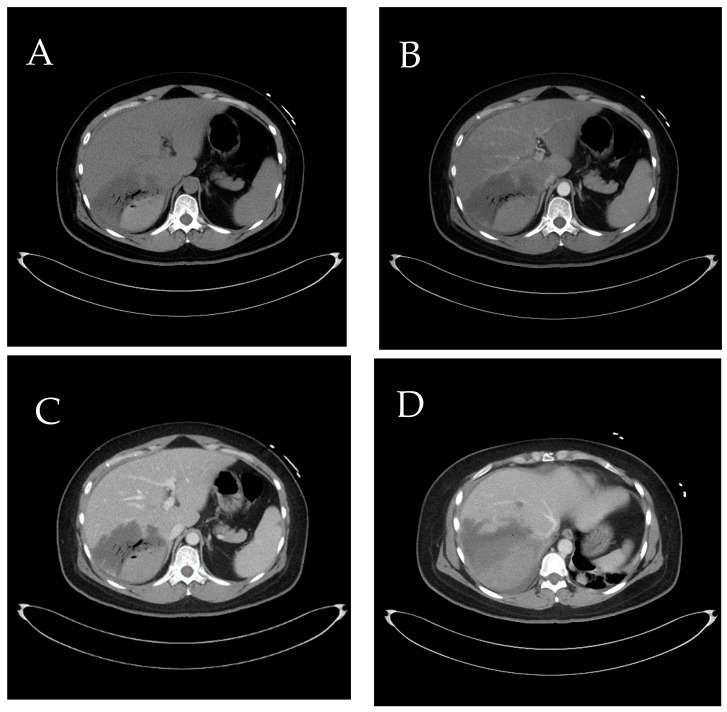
Four days following the initial TAE, a dynamic CT scan was conducted on the patient. (A–C) Representing the same anatomical level, precisely at the bifurcation height of the portal vein. (A) Pre-contrast phase. (B) Artery phase. (C) Portal phase. In comparison to the previous scans, an enlargement of the hematoma size is noticeable. Surrounding the hematoma, hypodensity and air are observed, suggesting necrotic changes induced by ischemia. (D) Depicting the bifurcation point of the left, middle, and right hepatic veins. The necrotic regions are still evident in this area.
